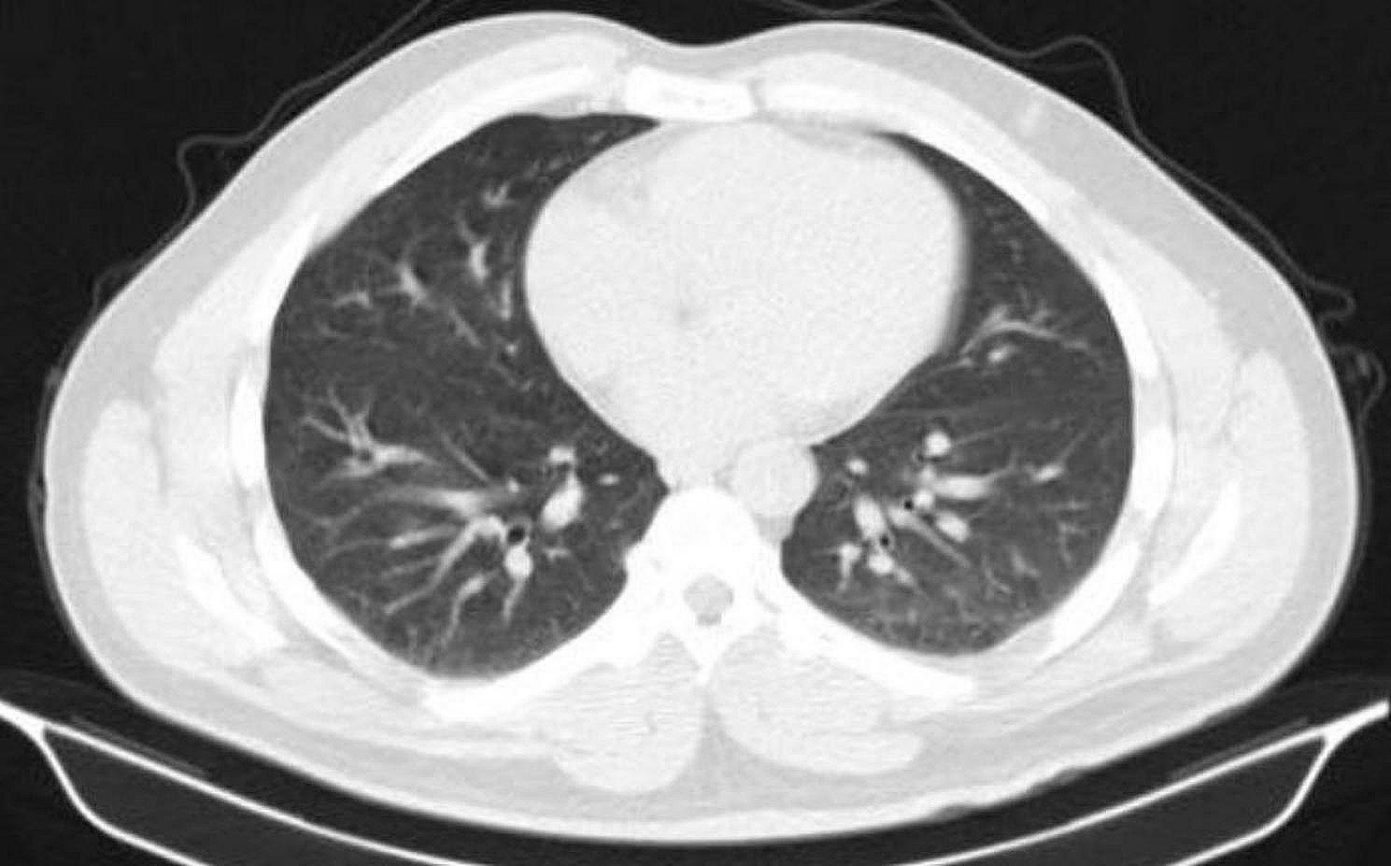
Lung CT scan of a 38-year-old man without underlying disease, normal
